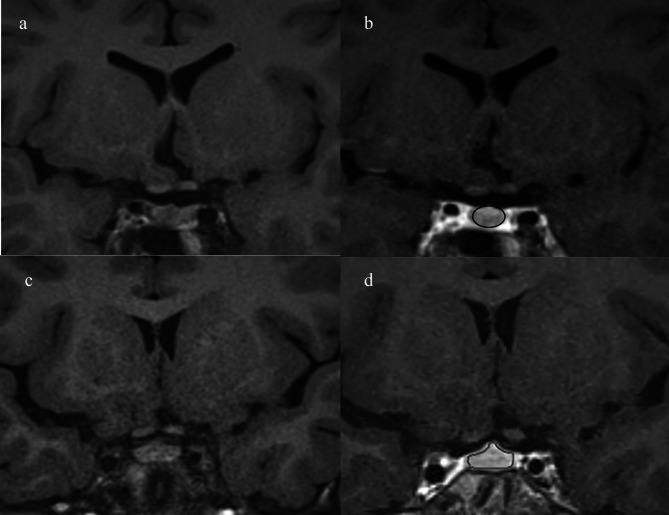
T1-FLAIR and Gd-enhanced MRI images of a 14-year-old male with severe GHD (a,b) and an 8-year-old male with normal GH function (c,d). The ROIs of the anterior pituitary gland were obtained at the maximum diameter in post-Gd-enhanced coronal T1-FLAIR images (arrow in b and d). The ratios of Gd-enhanced signals in the anterior pituitary gland to white-matter signals in the temporal lobe (signal intensity of the anterior pituitary gland / (signal intensity of the anterior pituitary gland + signal intensity of the right temporal lobe)) were 0.68 and 0.73, respectively
